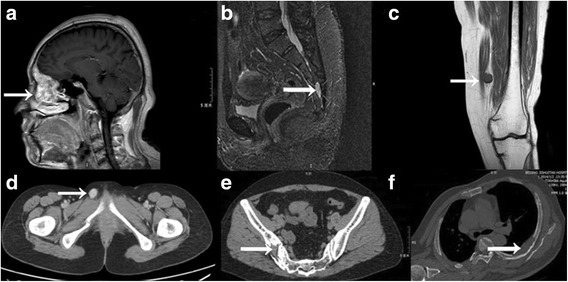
Magnetic resonance imaging (MRI) and computed tomography (CT) of causative tumors. (a) Patient 5. Cranial MRI showed a soft mass (arrow) in the left nasal cavity and ethmoid, frontal, and maxillary sinuses. (b) Patient 2. MRI showed a tumor (arrow) in the sacral canal. (c) Patient 4. MRI showed a tumor (arrow) in the soft tissue of the left thigh. (d) Patient 10. CT showed a neoplasm (arrow) in the mons pubis. (e) Patient 3. CT showed a mass (arrow) in the right ilium. (f) Patient 12. CT showed a tumor (arrow) in the left fifth rib
